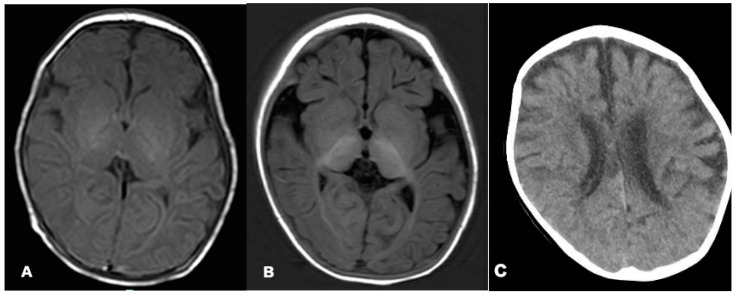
Brain MRI of the patient at the ages of 1 month (A) and 6 months (B). Brain CT of the patient at the age of 2 years and 10 months (C). Axial T1-weighted images suggested decreased periventricular white matter on both sides (A,B). Brain CT showed atrophic changes in both cerebral hemispheres (C).
